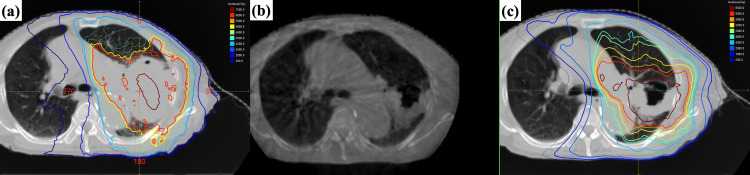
An example of adaptive radiation therapy performed for lung cancer (a) Dose distribution on the initial planning CT, (b) Cone-beam CT image at 44 Gy, and (c) Dose distribution on the replanning CT
